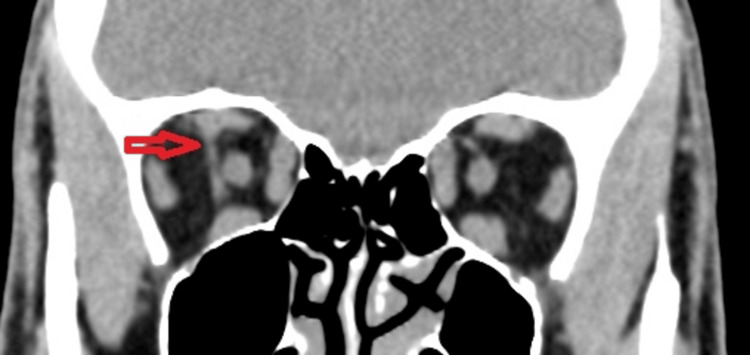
Coronal view of a plain computed tomography showing the orbit of the patient with an elongated tubular structure seen (red arrow) lateral to the right optic nerve extending from the superior rectus to the inferior rectus muscle
